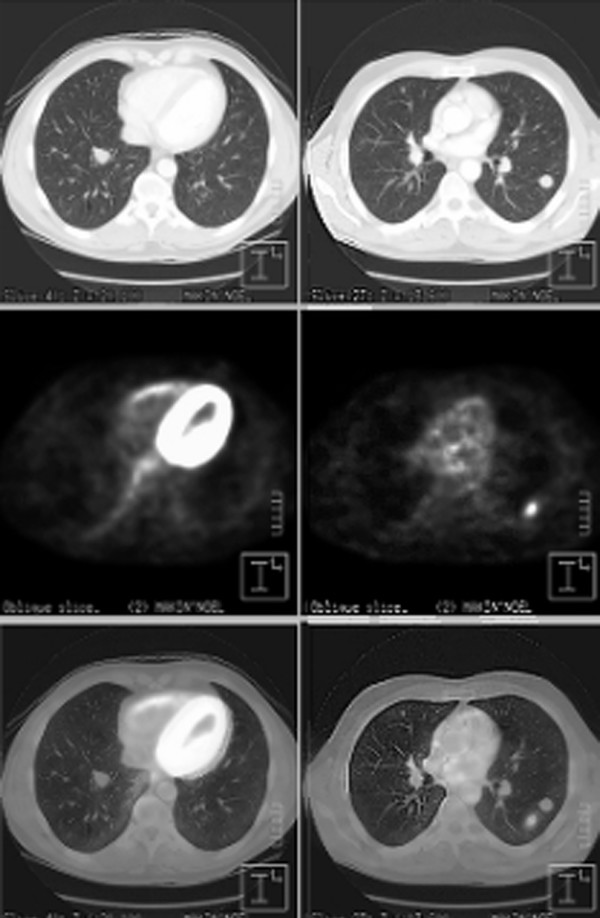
Top panel: *Computed tomography scan showing multiple rounded nodules in the right and left lung fields. *Middle panel: *Corresponding sections on PET scan. *Bottom panel: Superimposition *of PET scan on CT scan shows that the intense uptake on PET scan corresponds to the lesion in the left lower lobe on CT scan. The rest of the lesions in lung fields do not show any uptake on PET scan.
